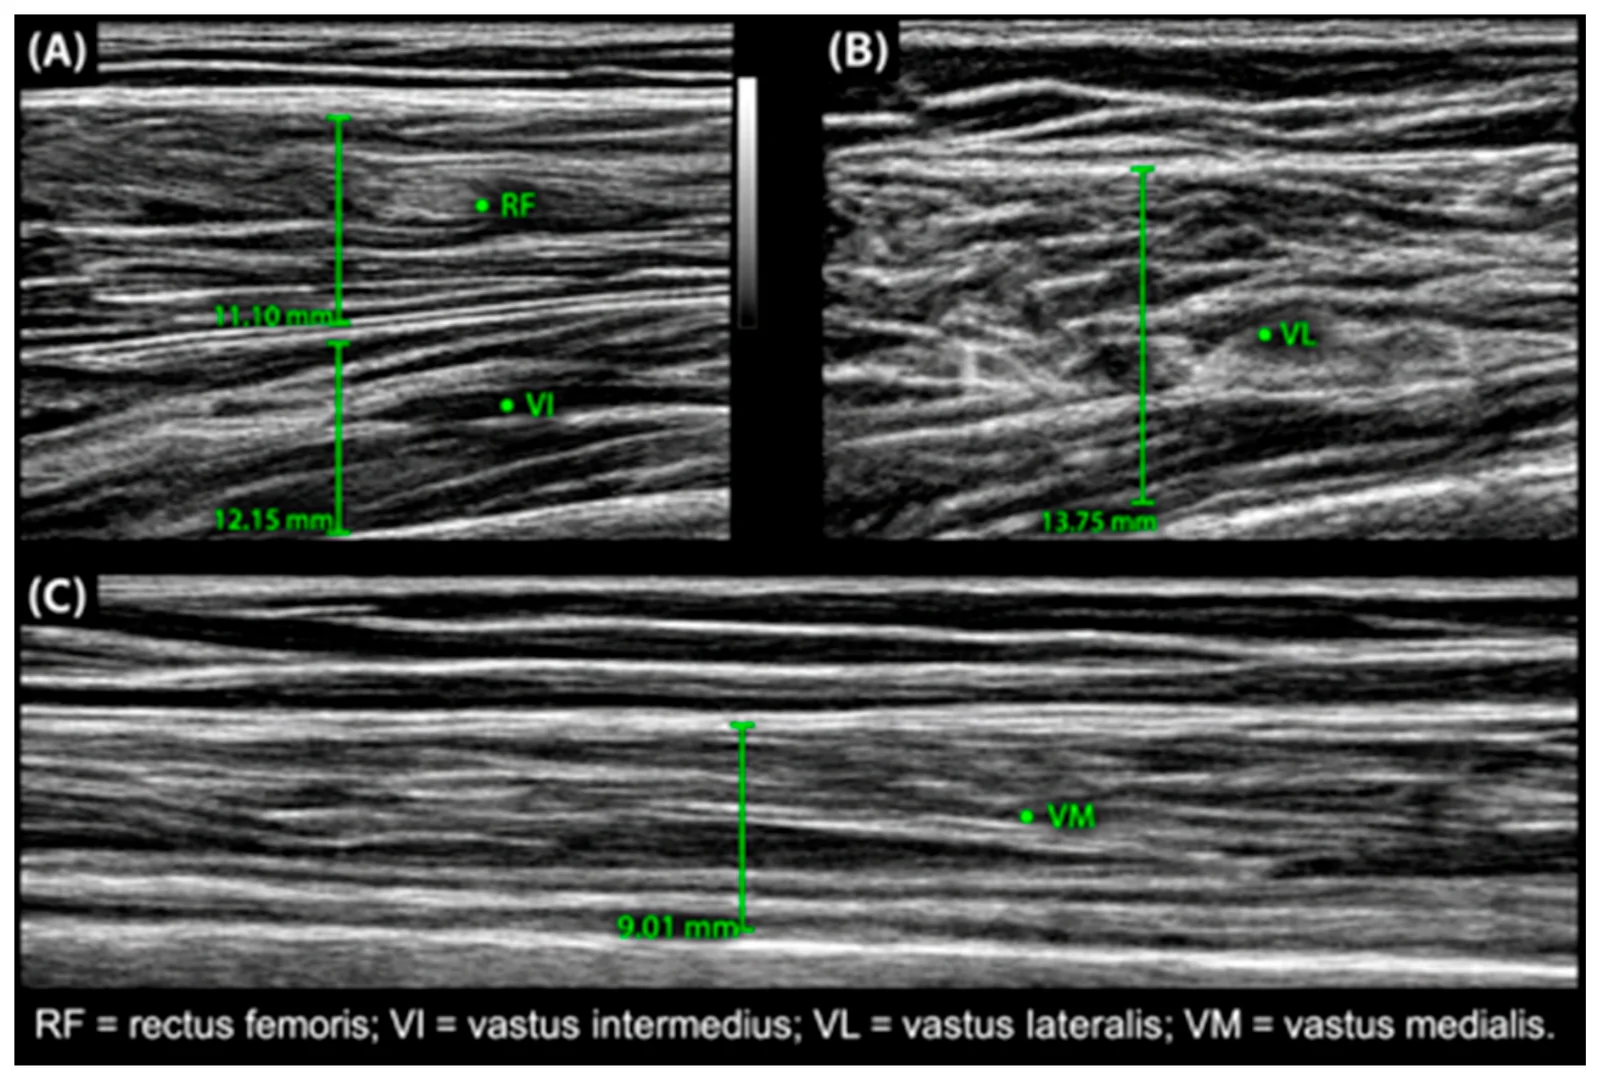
Representative RUSI images of quadriceps femoris muscle-thickness measurements. (A) Rectus femoris and vastus intermedius; (B) vastus lateralis; and (C) vastus medialis. Each panel represents a distinct ultrasound acquisition obtained at the corresponding anatomical measurement site. Muscle thickness was measured at the central vertical caliper line.
